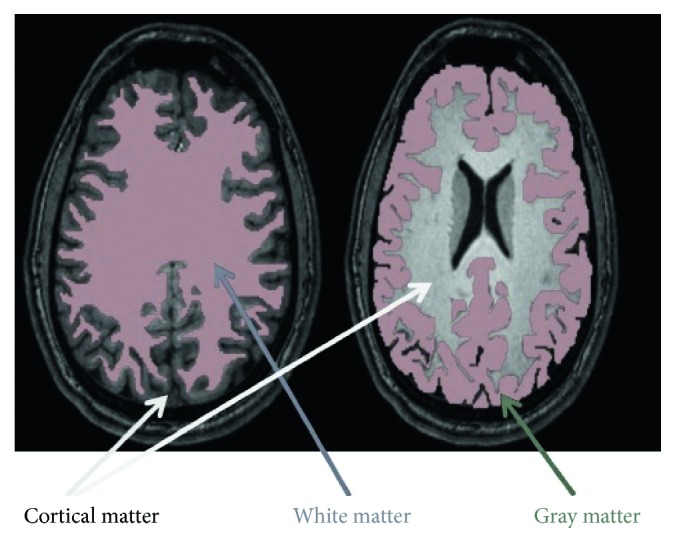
Sample manual segmentation (labeling) by neurologist expert of the gray and white matter regions in brain MRI images: white matter region (left) and gray matter region (right).
